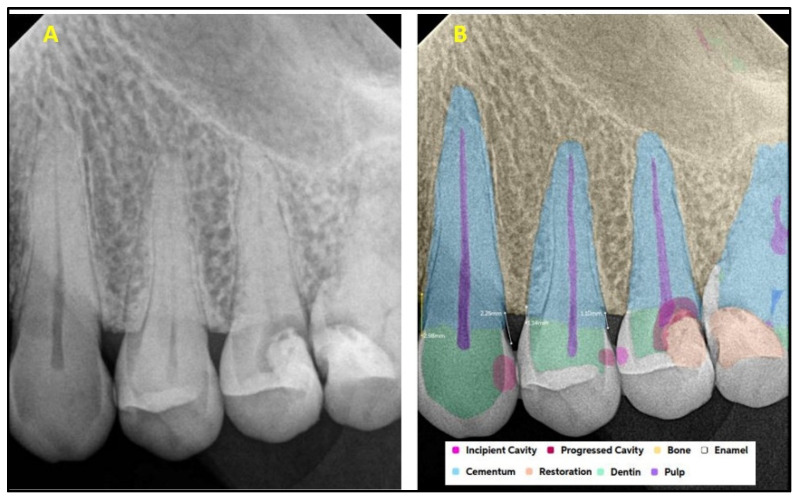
(A) Intraoral periapical radiographic image of maxillary posterior teeth; (B) analysis made by artificial intelligence software depicting diagnosed conditions (dental caries, restorations, and bone loss).
